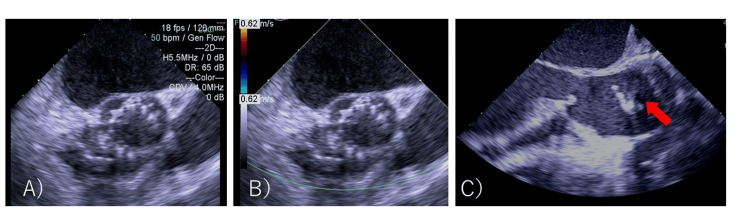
Transesophageal echocardiographic images of the second TAVR valve A: Mid-esophageal short-axis view (B mode) of the TAVR valve (B mode) showing that the second valve is normally implanted B: Short-axis view of the TAVR valve revealed no perivalvular regurgitation detected with color Doppler imaging C: Mid-esophageal aortic valve long-axis view after aortic clamping revealing the anterograde migration of the second valve due to surgical compression. A red arrow indicates the migrated second valve. The first valve is not visible because it has dropped out of the image into the LV due to loss of cardiac output. TAVR: Transcatheter aortic valve replacement, LV: Left ventricle
